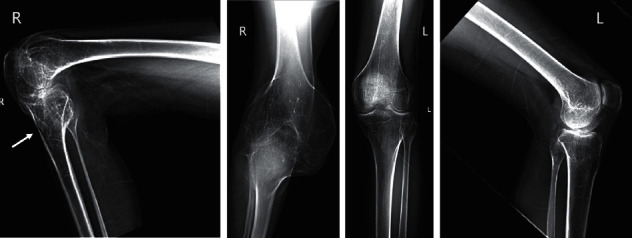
X-ray photography on both knees of Patient 3 before amputation.
